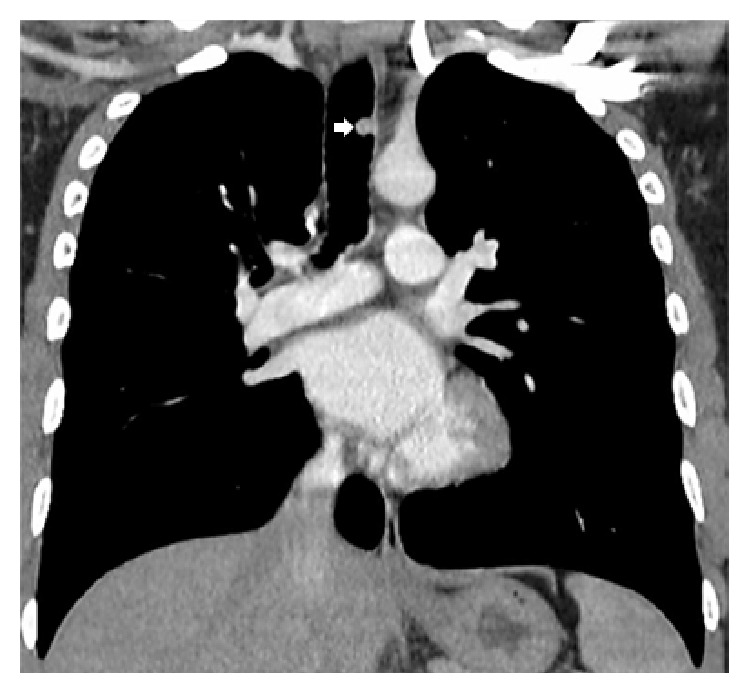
Computed tomography showed a homogeneously contrast enhanced polyp (white arrow) on the left lateral wall of the mid-trachea.
